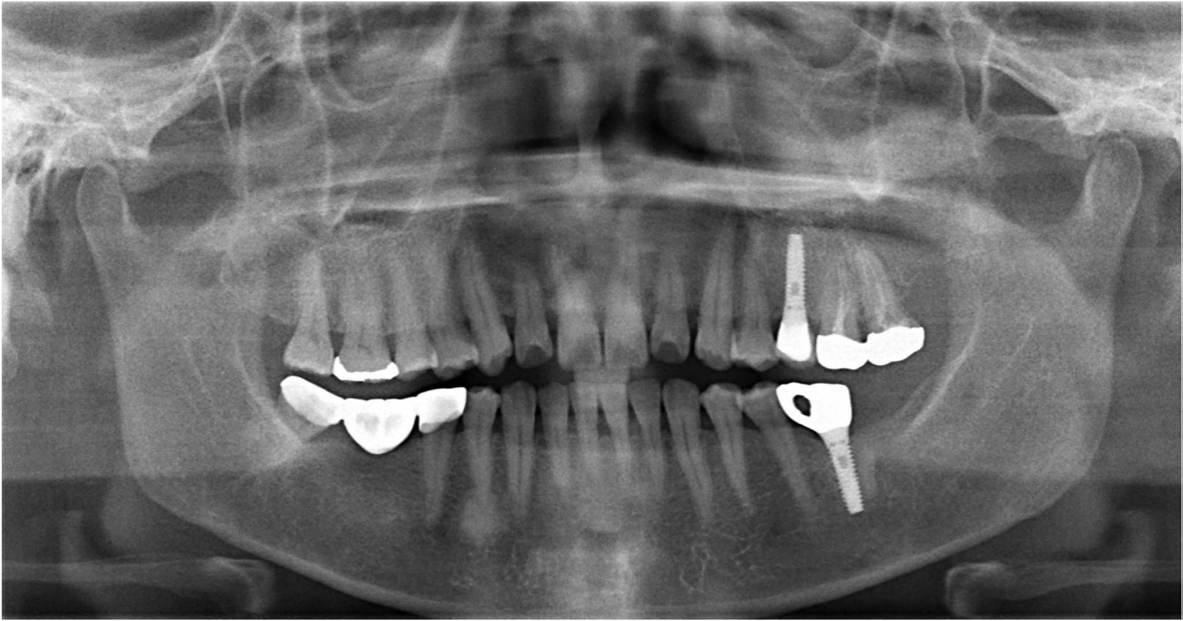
The panoramic radiograph of a 64-year-old female patient with odontogenic keratocyst (OKC) in left maxilla
